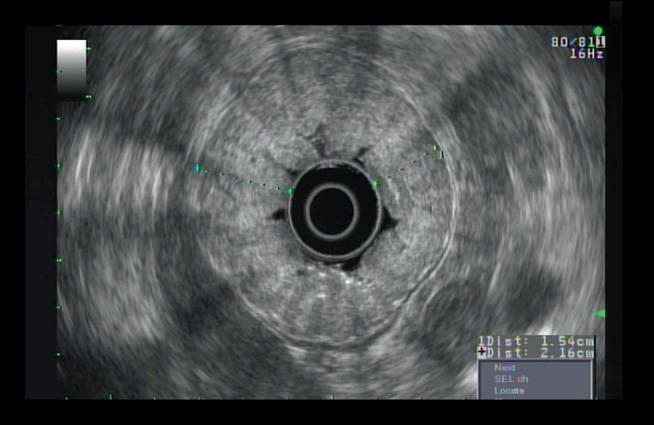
Endoscopic ultrasound showing wall thickening was seen in the body of the stomach. The thickening appeared to be primarily within the sub mucosa (Layer 3), >1 cm.
